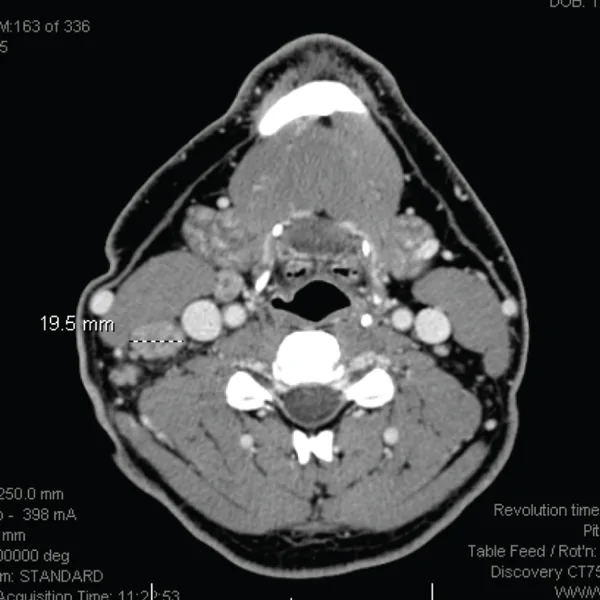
CT soft tissue neck showing the largest lymph node measured in the coronal plane [3].
